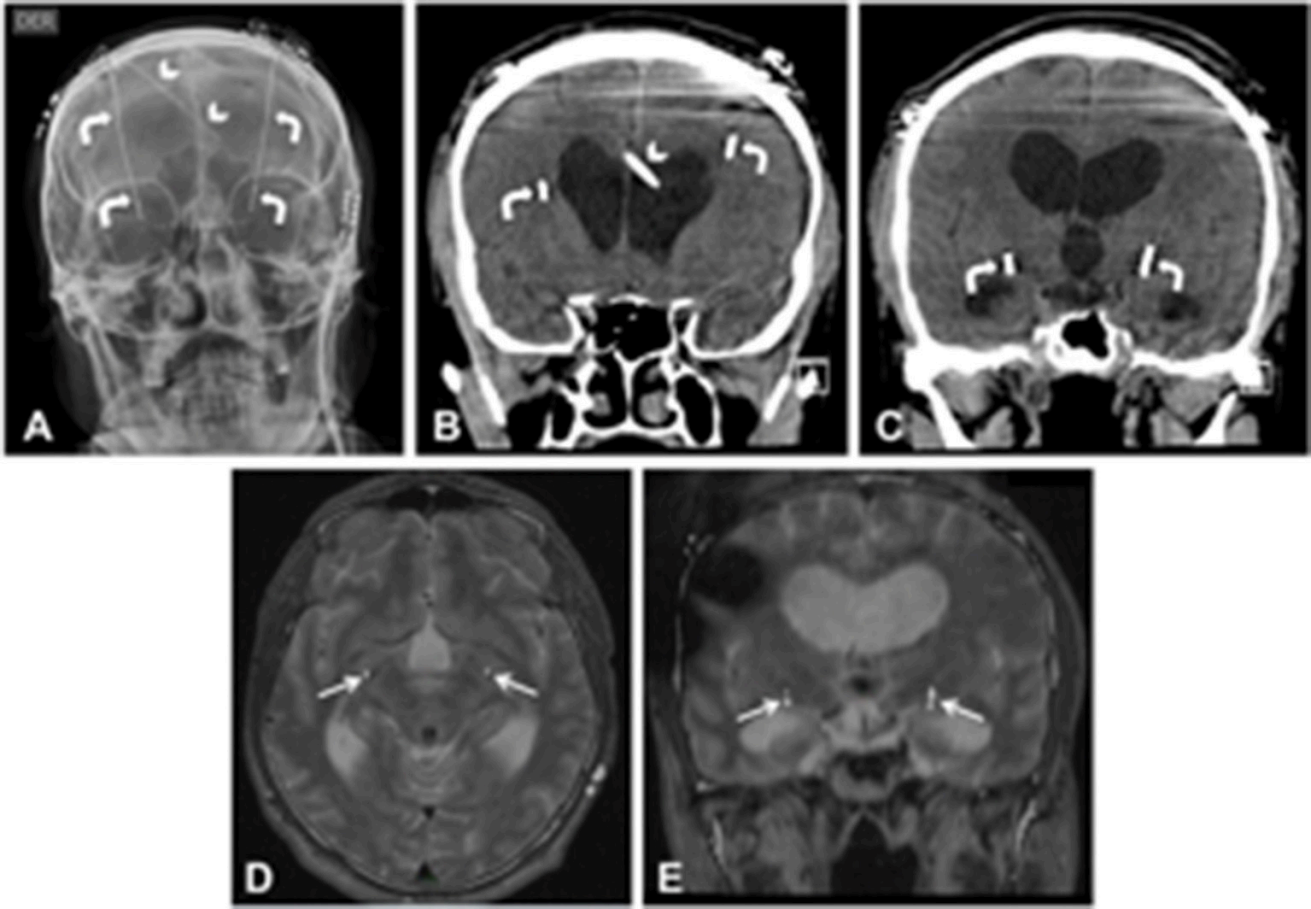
Postoperative GPi-DBS exams (A: skull radiography frontal view; B and C: brain CT coronal slices). The lead tracts (white curved arrows) avoid the right ventriculoperitoneal shunt catheter (white arrowheads) and the enlarged frontal horns of the lateral ventricles. Superimposed fused images between preoperative T2 MRI and postoperative CT (D: axial; E: coronal) confirmed the lead placement on the GPi bilaterally (white straight arrows).
